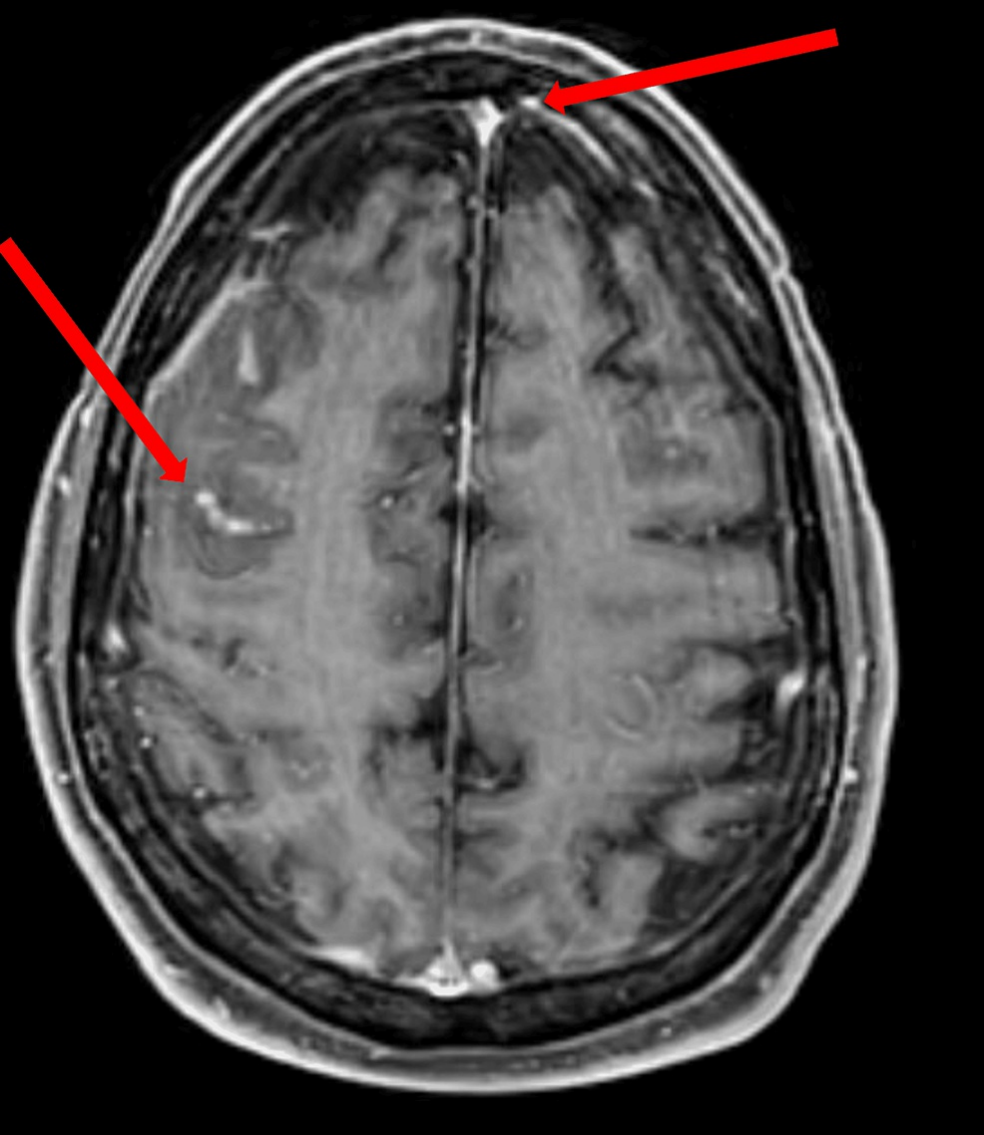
A T1 post-contrast MRI brain demonstrates pachymeningeal leptomeningeal enhancement as well as areas of cortical edema (arrows).
